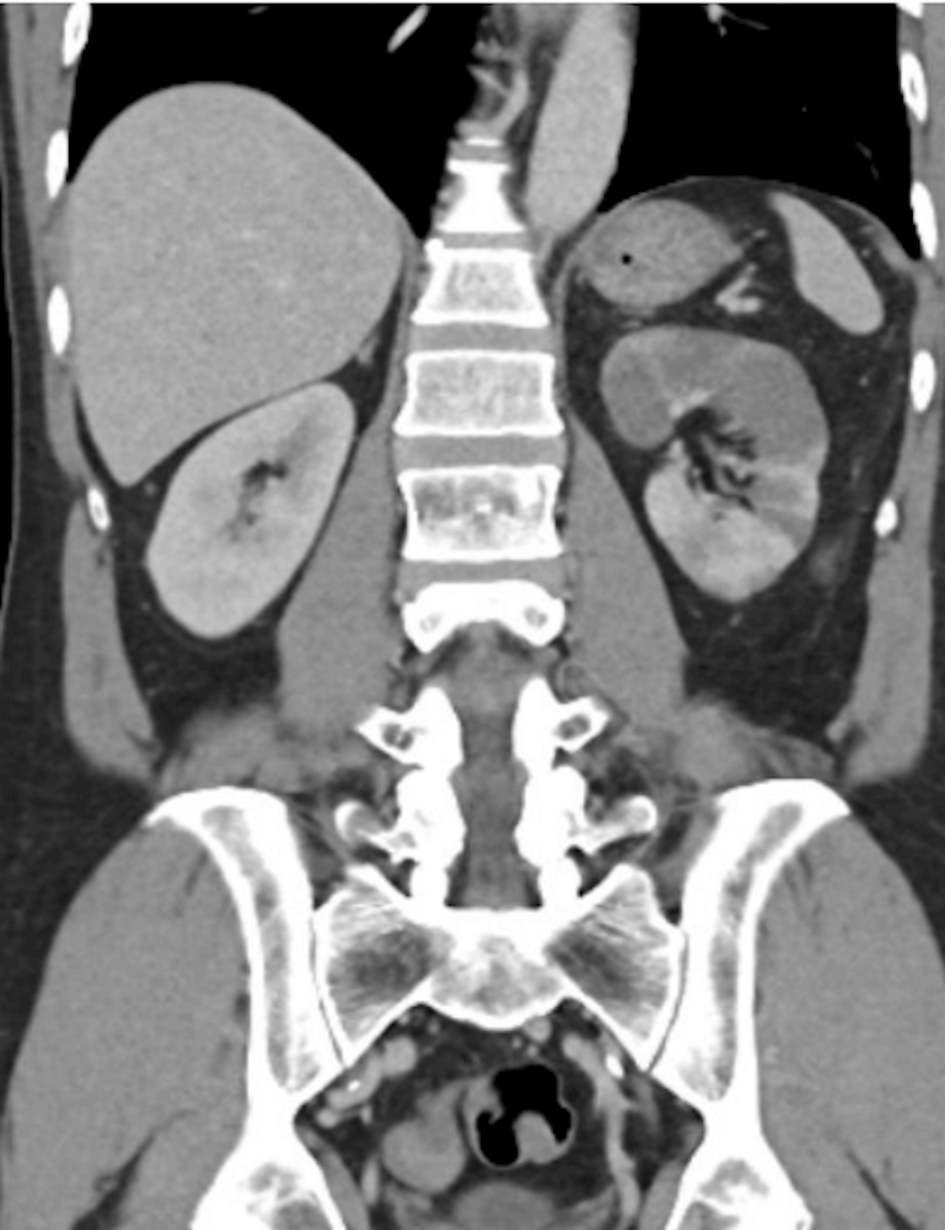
CT (coronal view) showing acute infarction of the superior pole of the kidney with wedge-shaped perfusion defect secondary to thrombosis of variant superior polar artery
